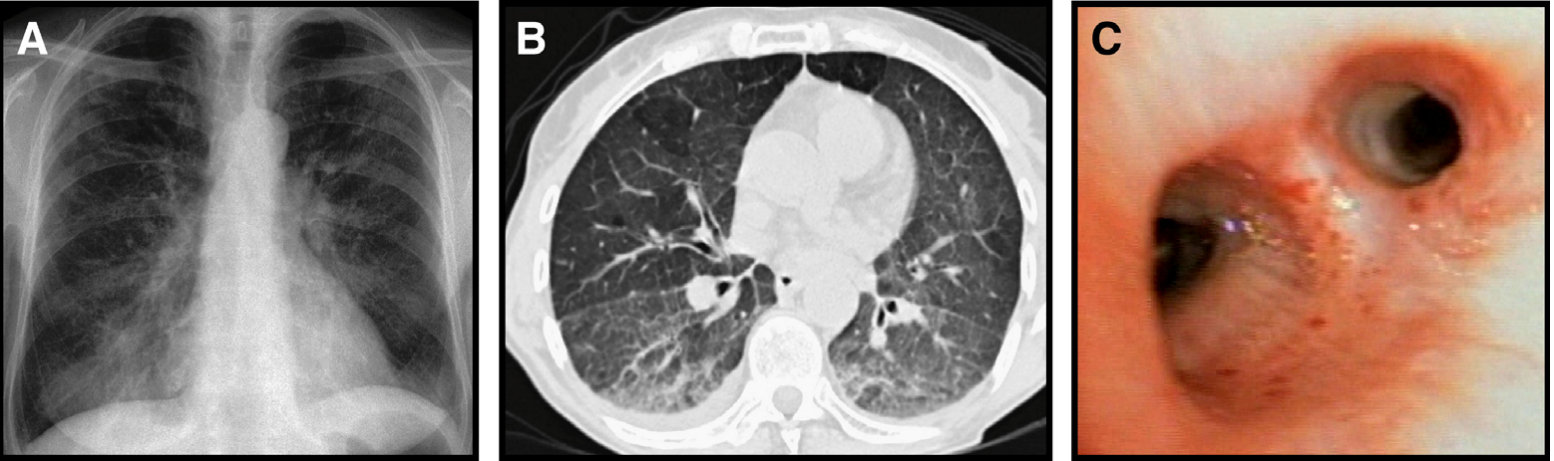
Imaging study from Case 1. A: Conventional chest radiograph with diffuse interstital pattern. B: High-resolution-CT (HRCT) featuring small pleural effusions and partial atelectasis of the right lower lobe in addition to distinctive ground-glass opacities. C: Fiberoptic bronchoscopy image showing a vulnerable tracheobronchial mucosa with multiple spontaneous bleeding stigmata, consistent with generalized tracheobronchial inflammation.
